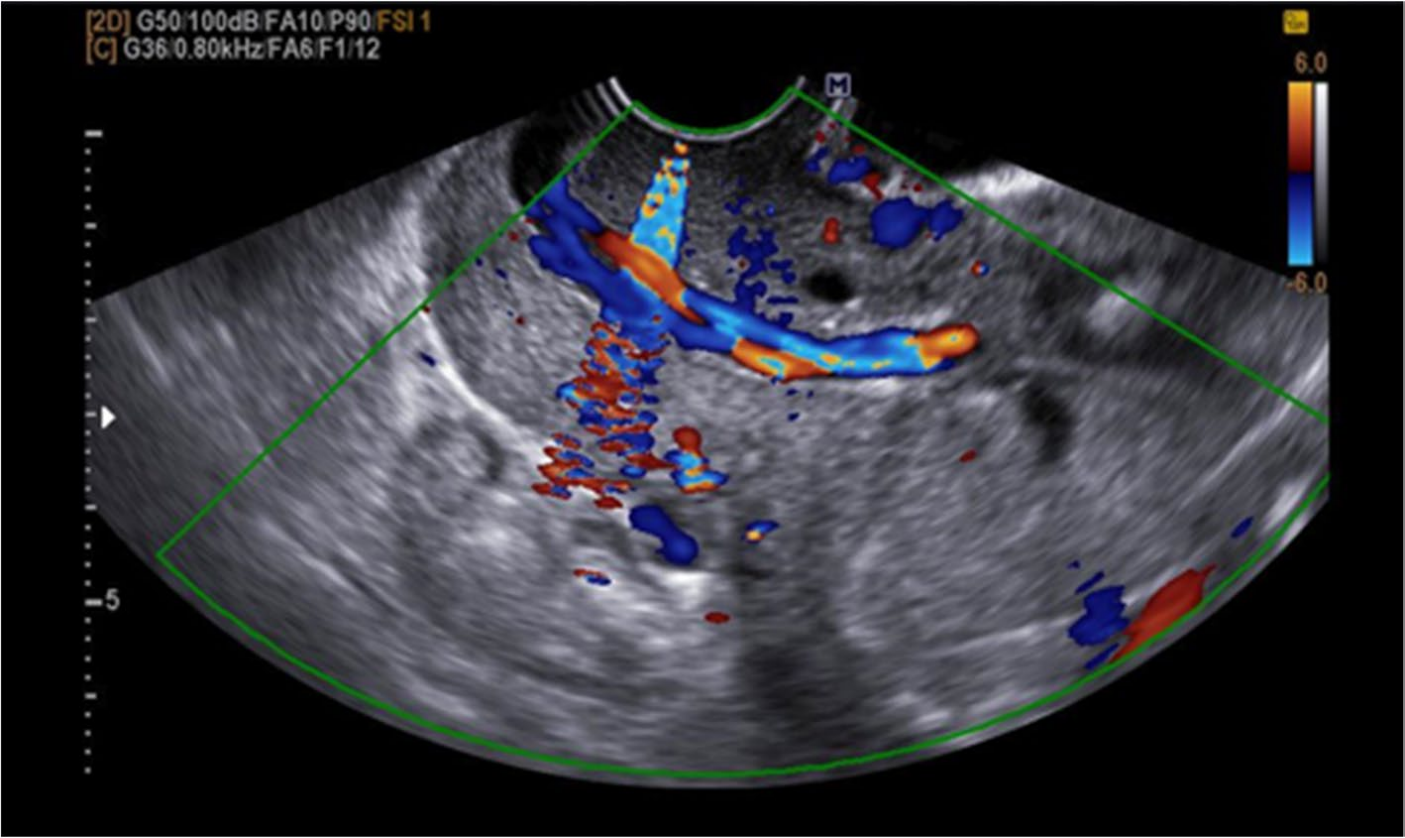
Color Doppler image demonstrating cord prolapse
